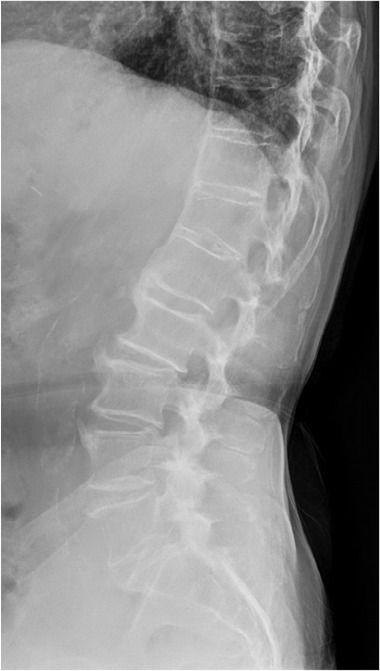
Lateral lumbar radiograph. There is bony bridging of the lower thoracic spine and T12-L1 bodies anteriorly. Bulky anterior syndesmophytes are depicted in the lumbar vertebrae form L1 to L4. However, there is no evident bridging except at L1–L2 level.
